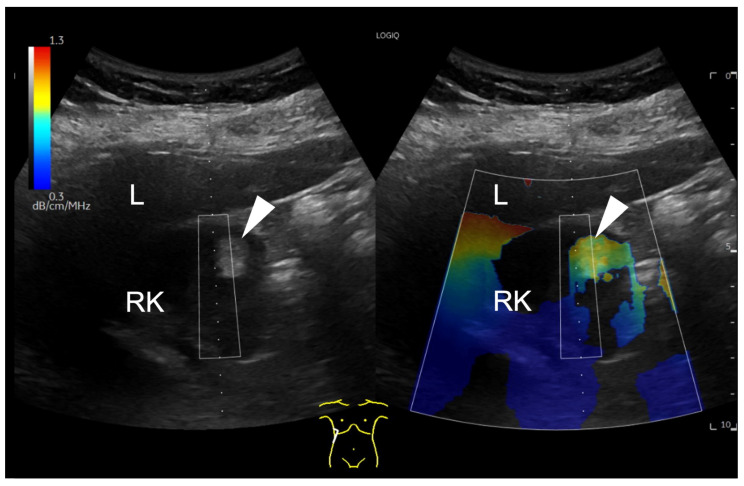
B-mode ultrasound image showing a focal hyperechoic lesion (arrowhead) in the cortex of the right kidney (RK). In the attenuation map on the right side, the lesion appears in red and yellow, representing a higher attenuation than the surrounding tissue. The level of attenuation is shown color-coded on the scale on the top left corner of the image. Next to the right kidney, the liver (L) is displayed. A region of interest (ROI) box is displayed above the hyperechogenic lesion.
